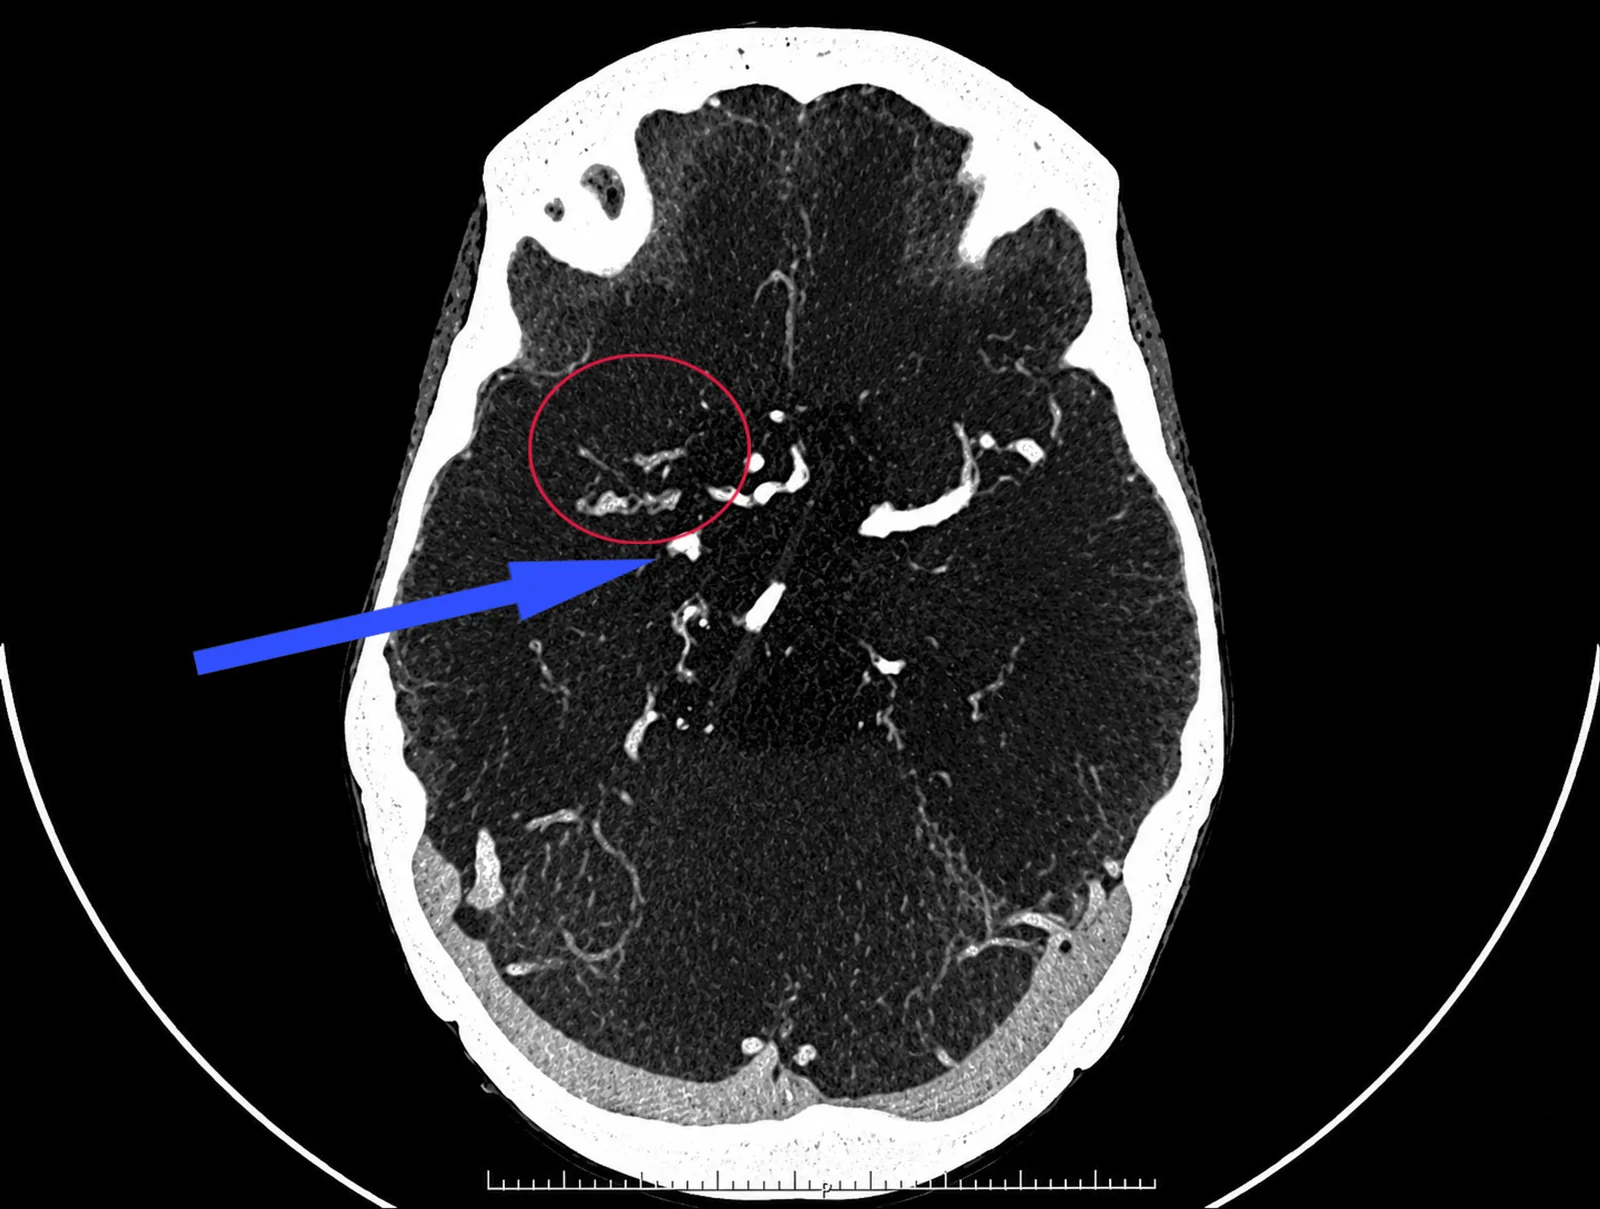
Carotid and cerebral computed tomographic angiography No contrast in the communicating segment of the right internal carotid artery, signifying complex occlusion (blue arrow) with formation of multiple collateral channels (red circle) along the circle of Willis on the right side.
